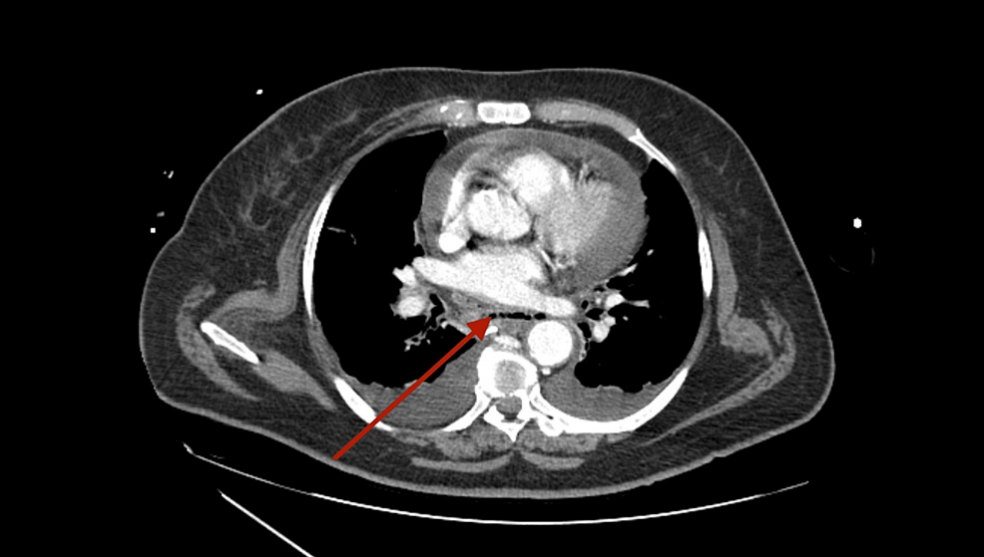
Pre-esophageal infra-carinal collection Contrast-enhanced thoracoabdominal CT showing a pre-esophageal infra-carinal collection with 4 mm maximum anteroposterior thickness and 50 mm in the largest transverse axis, containing liquid and gas bubbles (arrow) suggesting perforation of the esophagus CT: computed tomography
